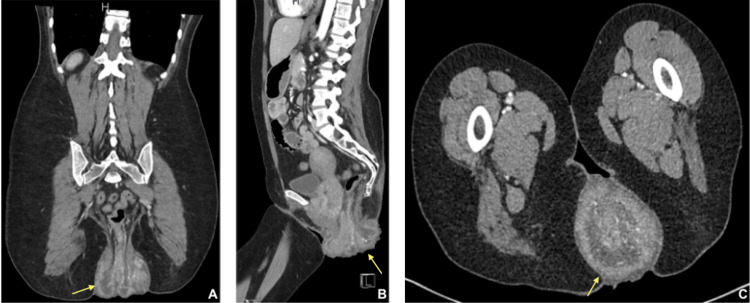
Preoperative computed tomography imaging with contrast, demonstrating a full-thickness, hyperdense rectal prolapse (yellow arrows), with edema and signs of ischemia. (A) Coronal view. (B) Sagittal view. (C) Transverse view.
